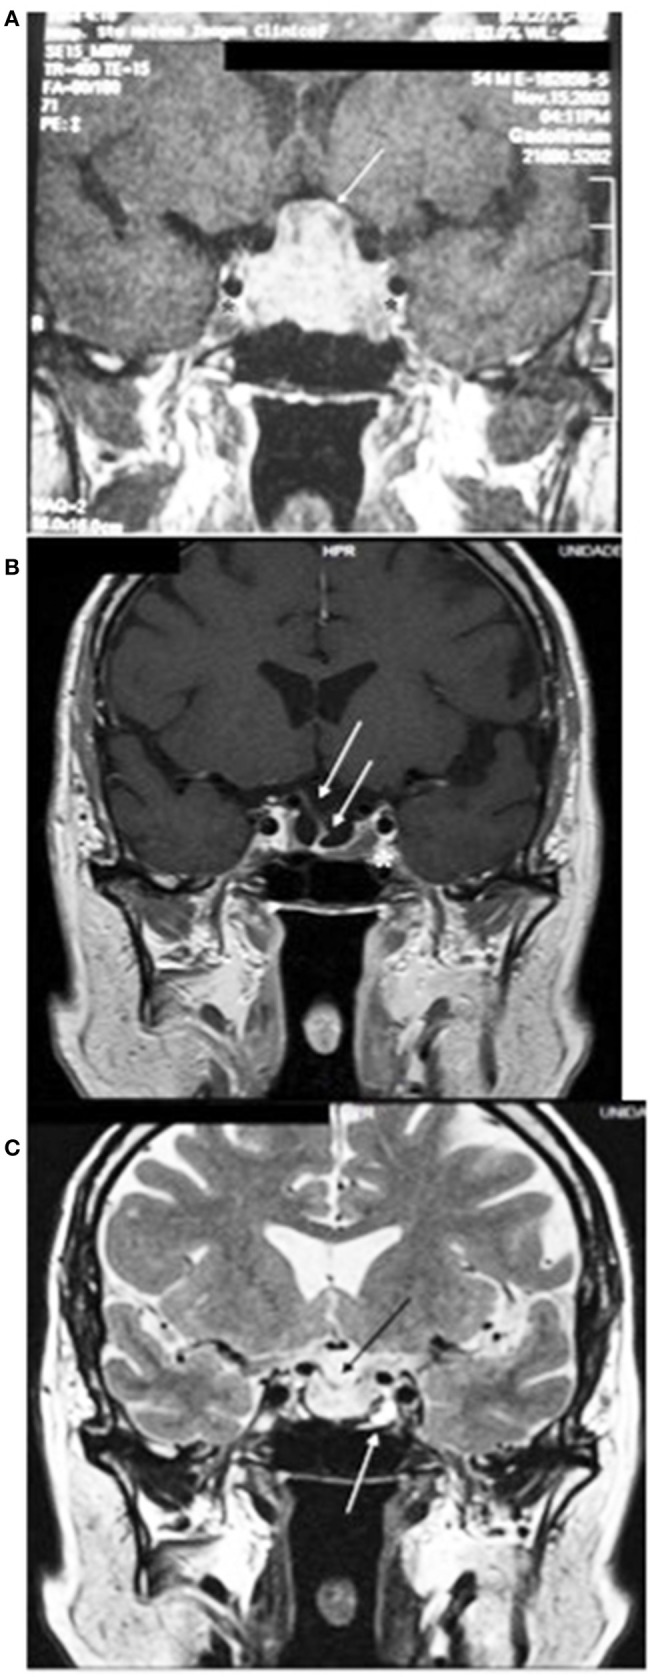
(A) Magnetic resonance imaging of the sella turcica before starting the treatment with cabergoline, on T1 coronal, with the tumor measuring 25 × 20 × 15 mm, with heterogeneous contrast, with cystic appearance, bilaterally invading the cavernous sinuses (^*^) and compressing the optic chiasm (white line). (B) After 14 years of treatment, with heterogeneous impregnation of the contrast medium, a small lesion is visible with a close association with the left carotid (^*^), invagination of the optic chiasm, and the pituitary stalk deviated to the right (white arrows). (C) T2 image shows a small lesion, with a hypersignal (white arrow), with cystic appearance, and invagination of the optic chiasm (black arrow).
